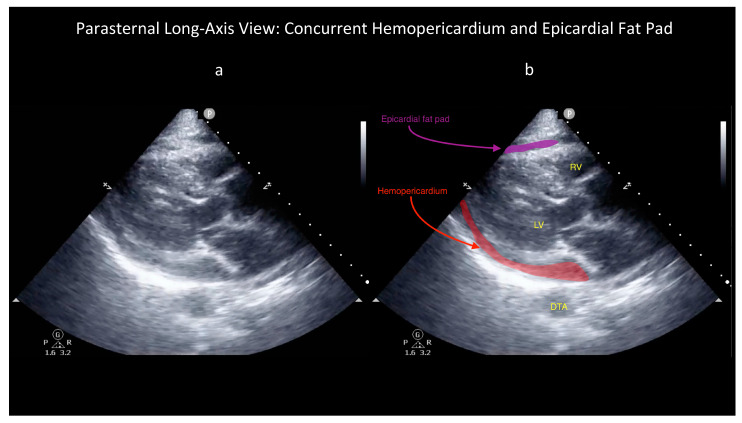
Unlabelled (a) and labelled (b) still image obtained as part of the same FoCUS exam that produced Figure 3 but now viewing the heart from the parasternal long-axis (PLAX) plane. Note the location of the epicardial fat pad anterior to the right ventricle (RV). Concurrently, this patient also had a moderate amount of hemopericardium visible in this view between the left ventricle (LV) and descending thoracic aorta (DTA) (see also Supplementary Video S4) whereas this blood is difficult to appreciate and/or differentiate from the patient’s epicardial fat in the subcostal four-chamber view (see Figure 3 and Supplementary Video S3).
